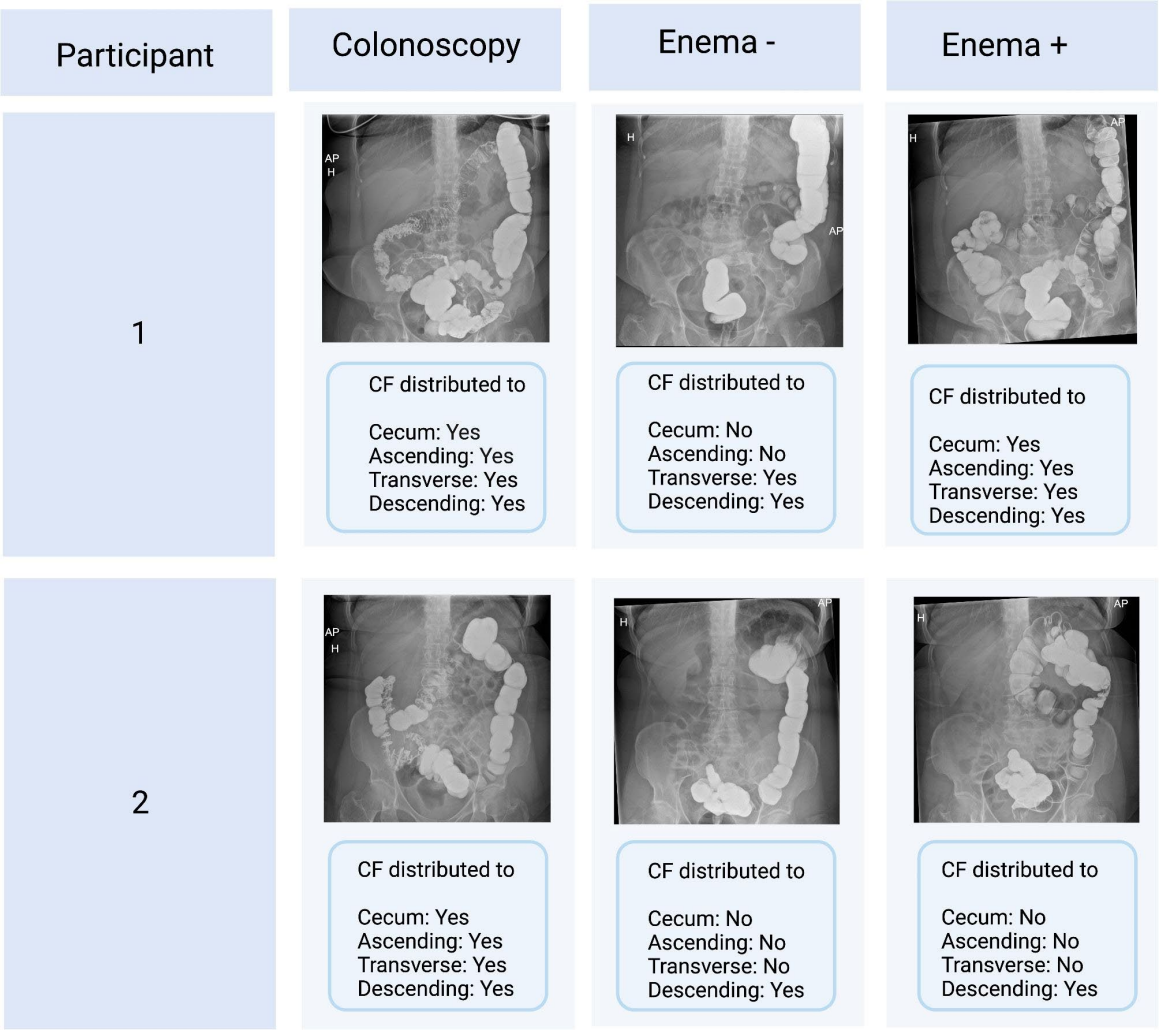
X-ray pictures from two study participants after colonoscopy, enema (-) and enema (+), created with BioRender.com
